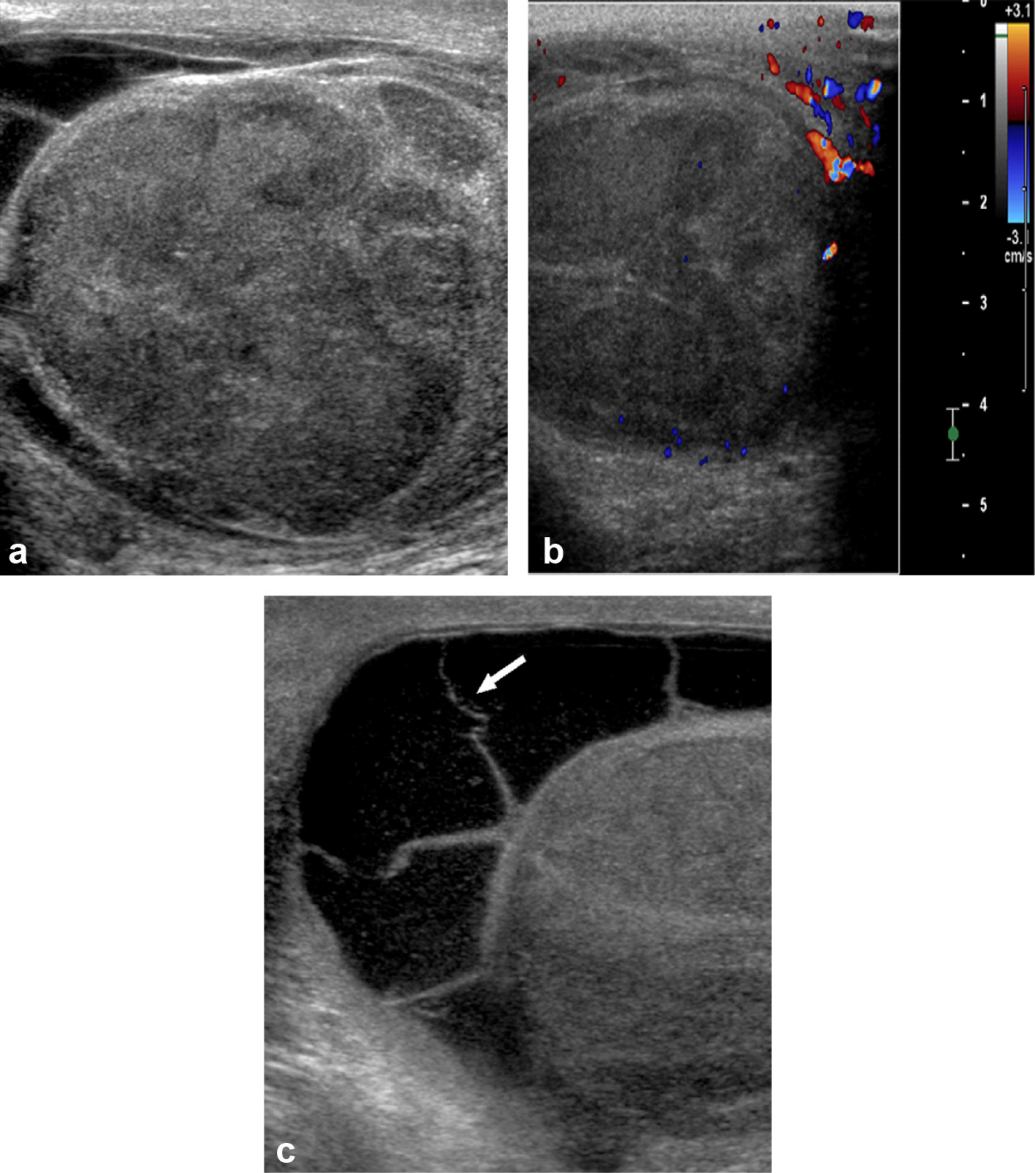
71-year-old male with scrotal pain and swelling. (a) Longitudinal sonographic
